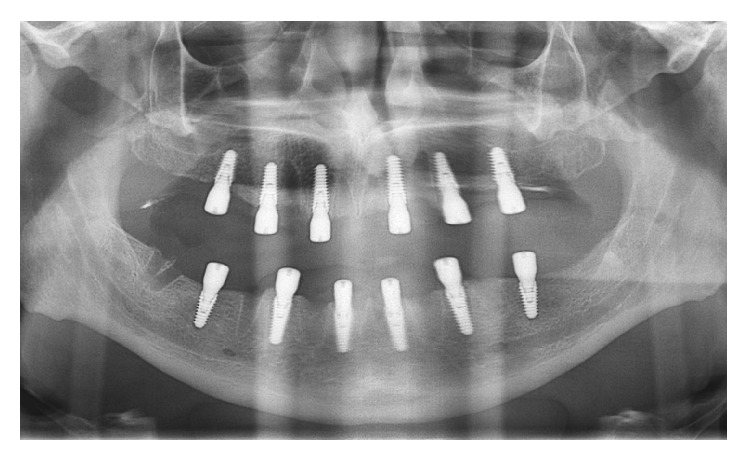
Panoramic radiograph taken immediately after implant placement.
